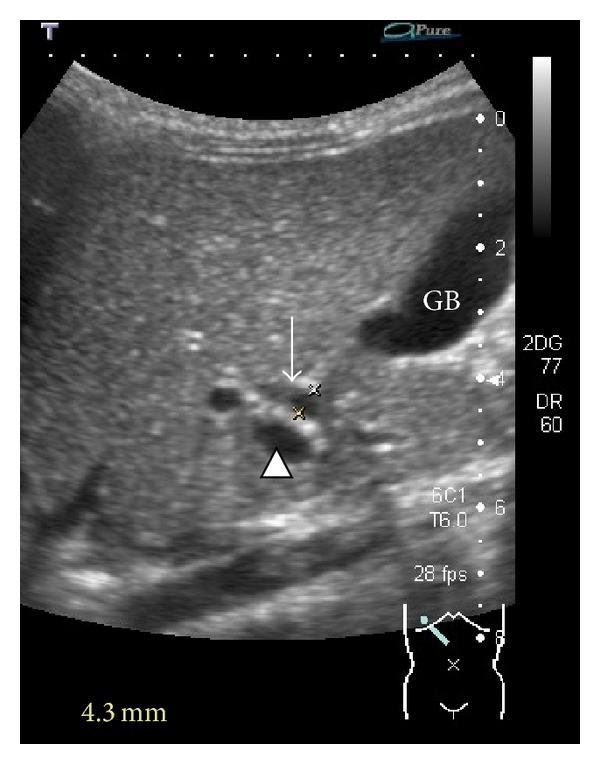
Common bile duct dilatation on ultrasound (arrow). The duct is measured as 4.3 mm. Portal vein (arrowhead). GB: gallbladder.
